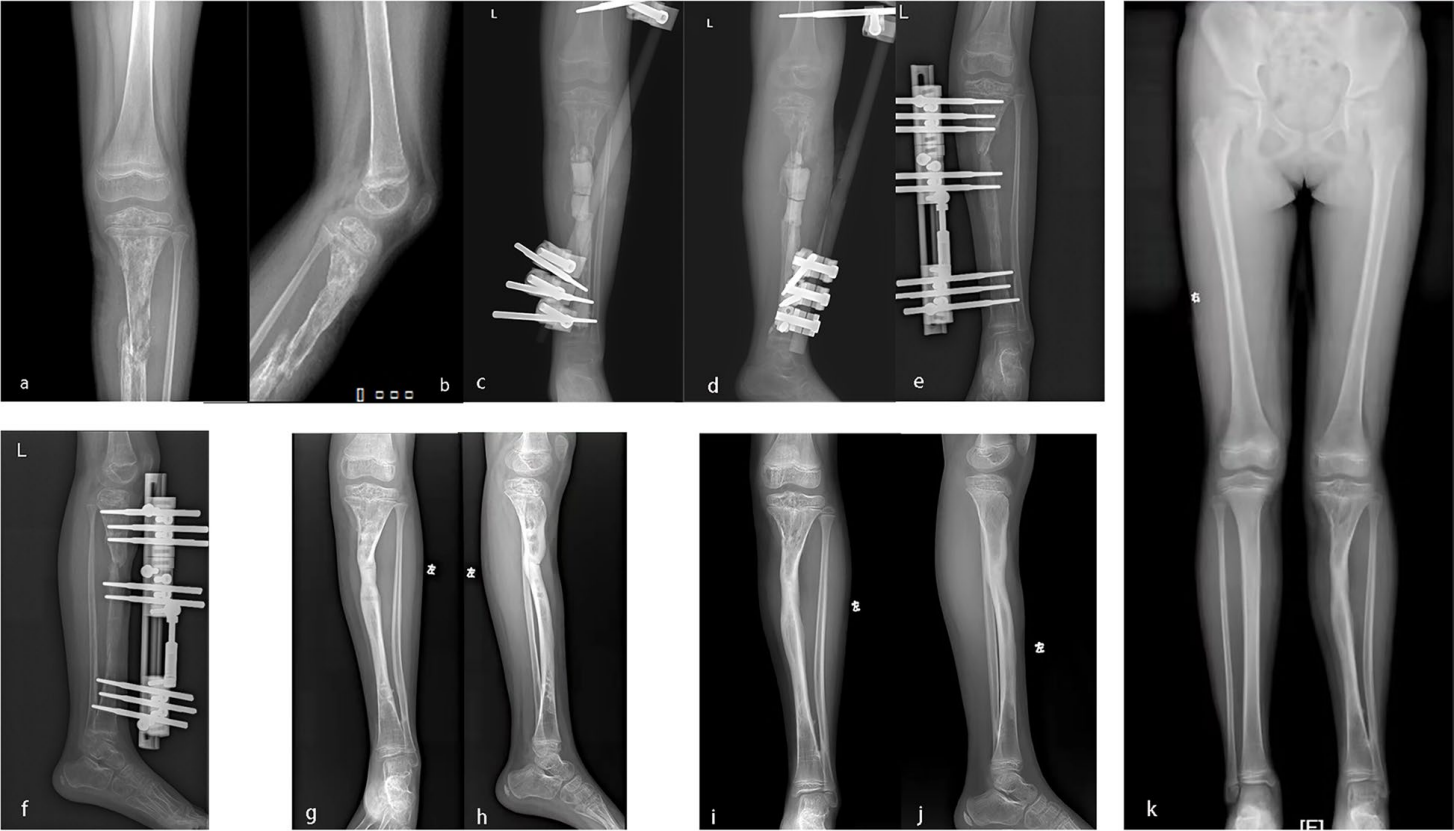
a-b: A six-year-old girl had suffered left proximal tibia HO. c-d: Vancomycin impregnated CS was placed in the void spaces left by decompression and debridement. The tibia was fixed by a temporary external fixator. e–f: A terminal unilateral external fixator was used for bone transport. Bone transport was finished 9 months postoperatively. g-h: External fixation was removed 18 months postoperatively. i-k: The latest X-ray was taken at 34 months postoperatively. The girl showed genu valgus deformity
